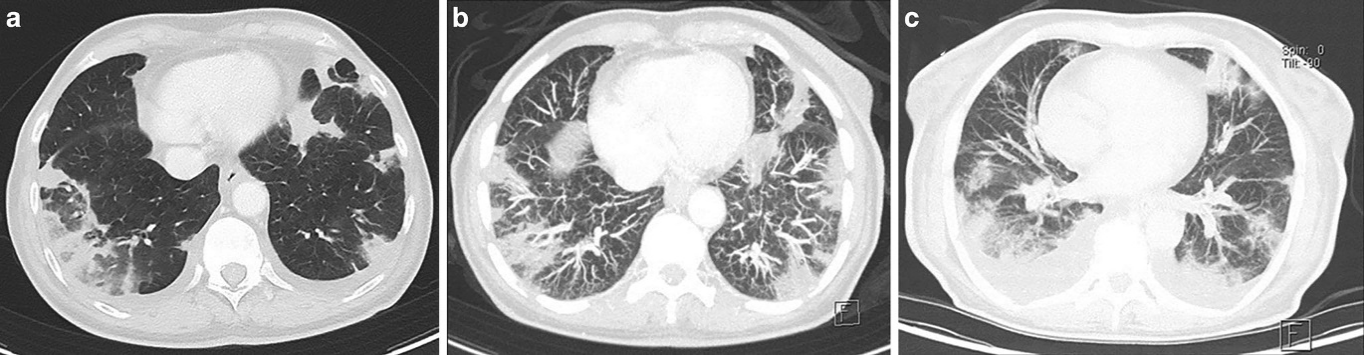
a First CT scan after initial diagnosis of a cardiac mass; b second CT scan about 2 weeks later; c third CT scan another 2 weeks later
